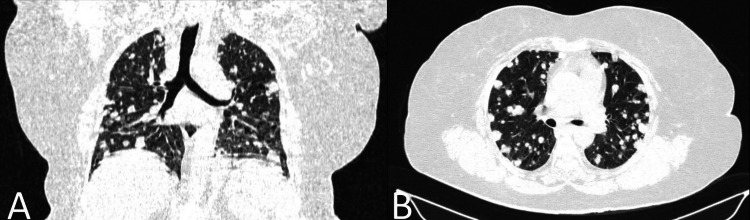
(A) Coronal section of HRCT-thorax revealing multiple variable-sized (2-20 mm diameter) soft tissue density nodules dispersed bilaterally throughout the lung parenchyma. (B) Axial section of HRCT-thorax displaying analogous widespread nodules indicative of metastatic lung disease. HRCT-thorax: high-resolution computed tomography of the thorax
